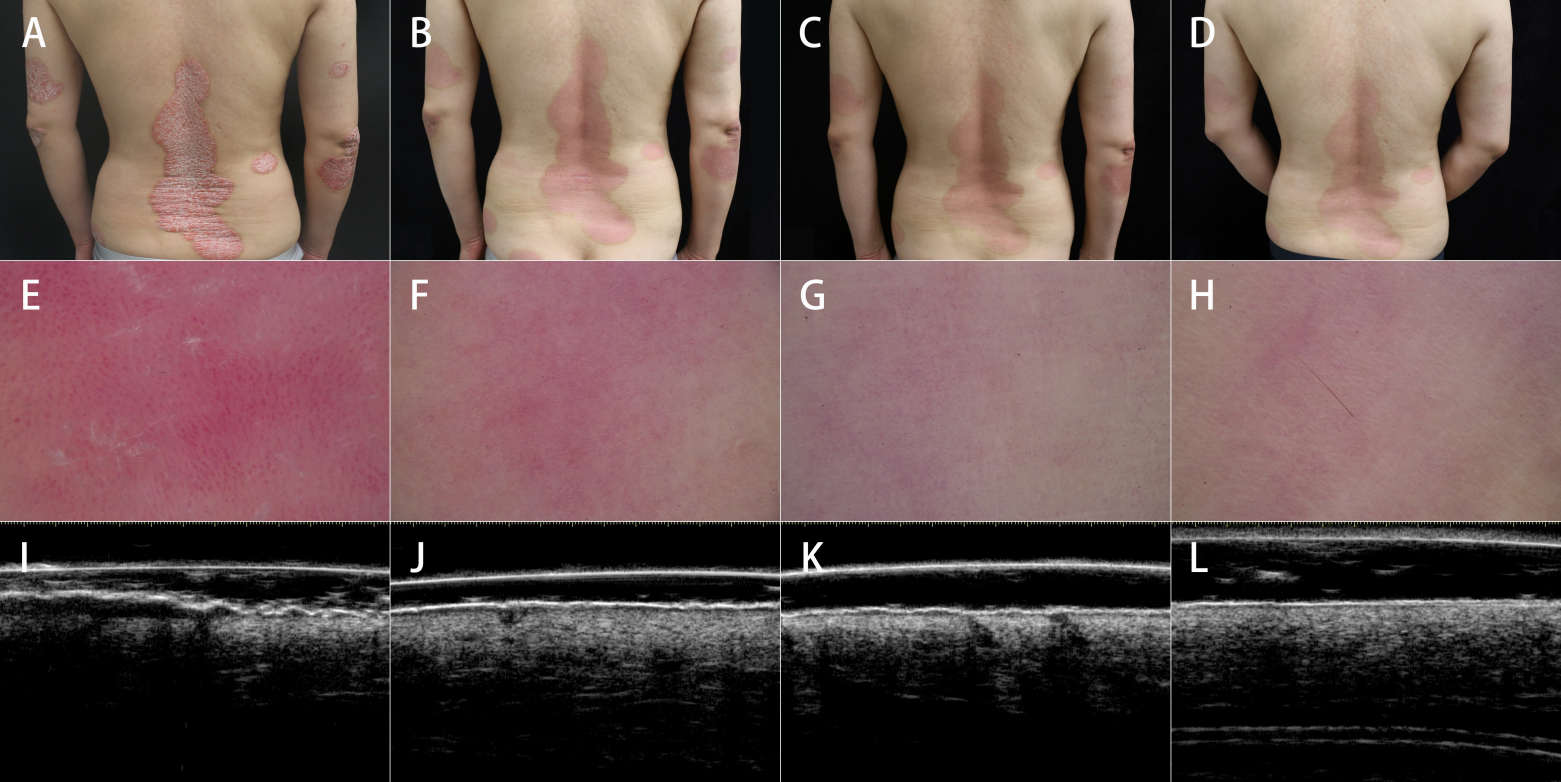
Clinical, dermoscopic, and ultrasonic assessments before and after treatment. Clinical assessment showed gradual improvements in erythema, scales, and induration from (A) baseline to (B) 4 weeks, (C) 8 weeks, and (D) 12 weeks after treatment. Dermoscopic assessment showed the normalization of the red background, vessel pattern, and scales and the appearance of linear vessels from (E) baseline to (F) 4 weeks, (G) 8 weeks, and (H) 12 weeks after treatment. HFUS showed significant improvements in the thicknesses of SLEB and superficial hyperechoic band from (I) baseline to (J) 4 weeks, (K) 8 weeks, and (L) 12 weeks after treatment, especially at the first 4 weeks of treatment.
